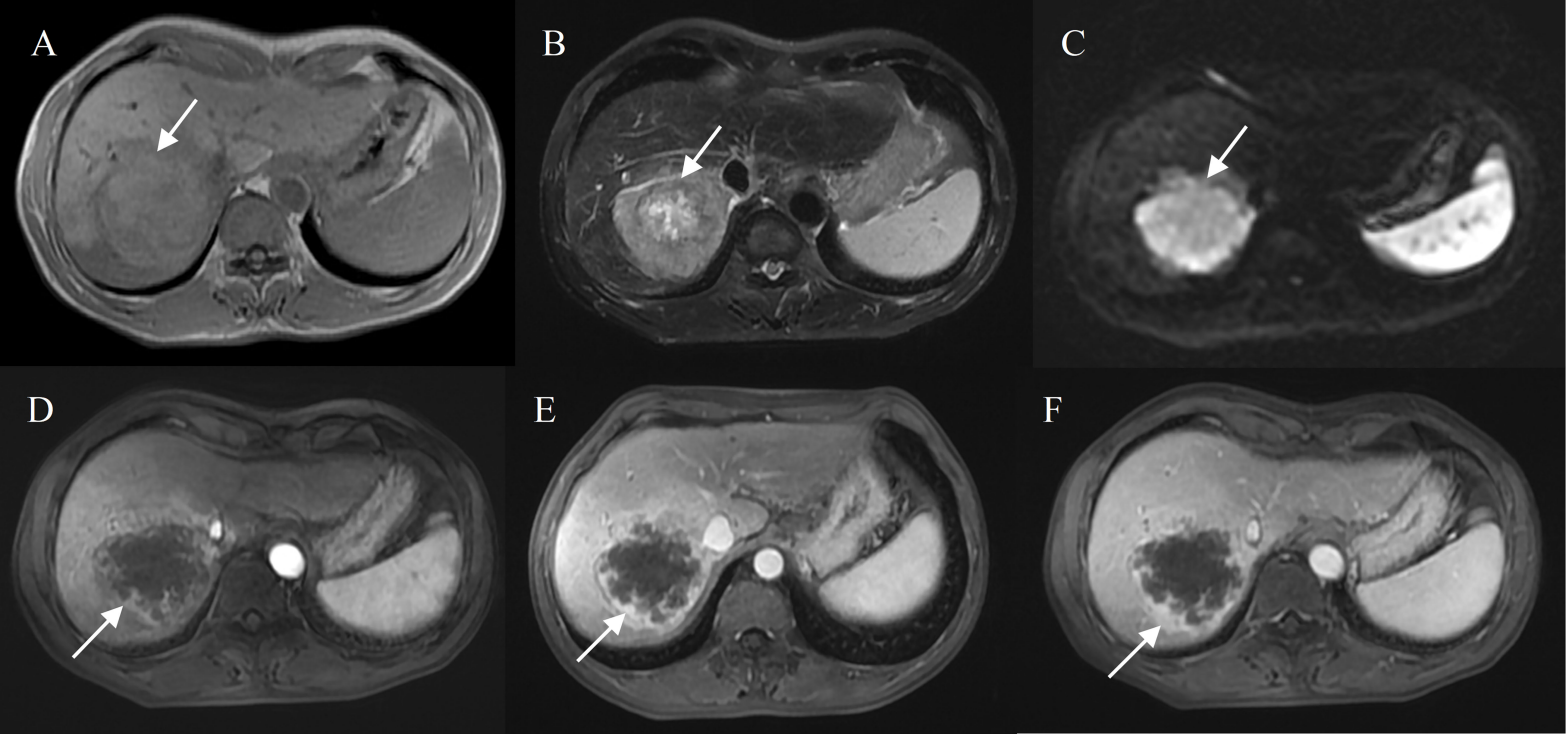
Hepatocellular carcinoma with peripheral rim enhancement in a 60-year-old male without chronic viral hepatitis. Gd-DTPA-enhanced MRI detected a round and non-smooth tumor (10.2 cm) with heterogeneous SI on T1WI and T2WI (A, B), restricted diffusion (C). Peripheral rim-like enhancement in the arterial phase (D) and sustained rim enhancement in the portal and delayed phase (E, F). Incomplete capsule enhancement on portal venous phase (E) and delayed phase images (F).
